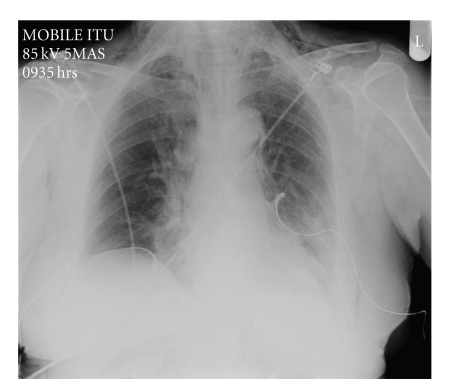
Chest X-Ray prior to chest drain removal.
